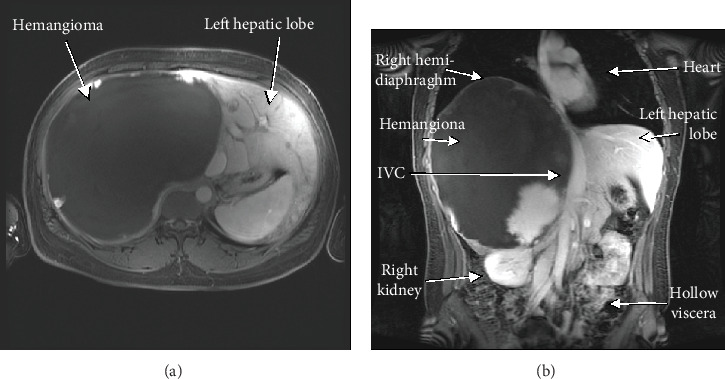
Magnetic resonance imaging of the abdomen. (a) Axial image showing massive T2 hyperintense lesion occupying a majority of the right hepatic lobe. (b) Coronal section showing the giant hemangioma displacing the hepatic hilum, left hepatic lobe, inferior vena cava, right kidney, right hemi-diaphragm, heart, and all the hollow viscera.
